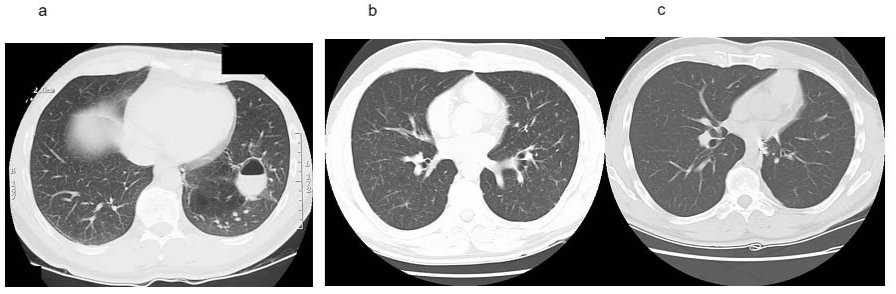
HRCT appearance of left lower lobe cyst, MAC-associated pneumonitis, and subsequent resolution. (a) Left lower lobe cyst with air-fluid level and adjacent infiltrate at initial presentation. (b) Bilateral, diffuse miliary nodular pattern six months later. (c) Resolution of miliary pattern after 3 months of antimycobacterial treatment.
